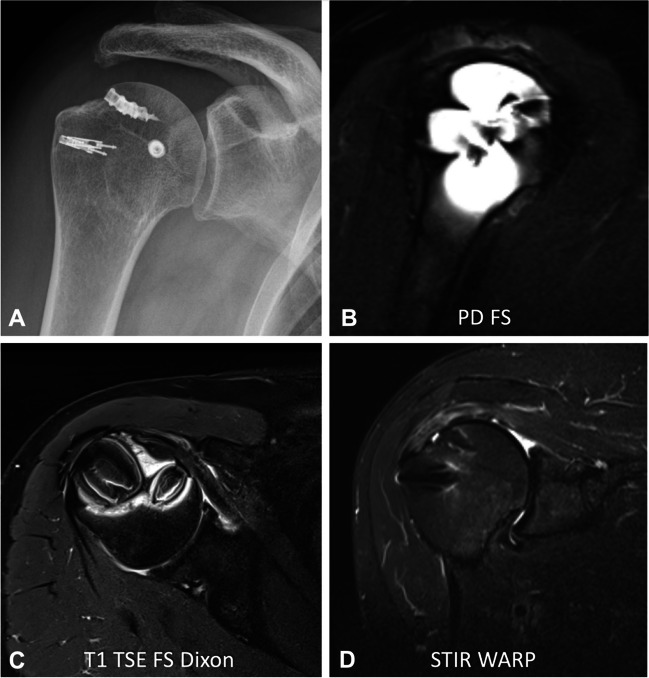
A 61-year-old patient after rotator cuff repair. The anteroposterior radiograph (A) shows four suture anchors in the humeral head made of titanium. The standard sagittal fat-saturated proton density (PD)-weighted image (B) shows the typical four-leaf clover artifact caused by the ferromagnetic metal implants, which interferes with the diagnostic evaluation of the humeral head and the insertion of the rotator cuff tendons. The axial T1-weighted Dixon MR sequence (C) demonstrates typical artifacts caused by the ferromagnetic metal implants in Dixon sequences. A dedicated short-tau inversion recovery (STIR) turbo spin echo sequence with optimized inversion pulse (STIR WARP) (D) shows significantly less artifacts with adequate fat suppression, allowing assessment of the integrity of the rotator cuff insertion. The images were acquired at 1.5 T with following sequence parameters: PD FS—echo time (TE) 58 ms, repetition time (TR) 3370 ms, receiver bandwidth 435 Hz/pixels; T1 TSE FS Dixon—TE 36 ms, TR 3620 ms, receiver bandwidth 250 Hz/pixel; STIR WARP—TE 47 ms, TR 4000 ms, receiver bandwidth 300 Hz/pixel, inversion time (IT)170 ms
